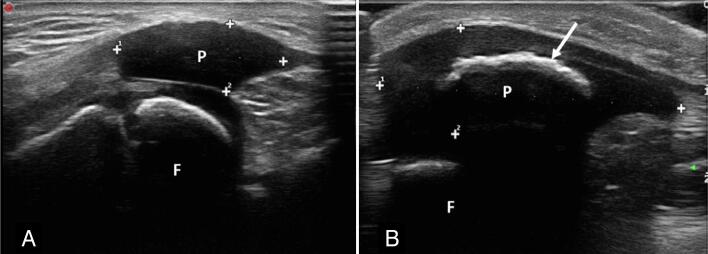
Male patient, aged 6 years and 9 months, with a previous diagnosis of right fibular hemimelia, complete agenesis of the fibula, and shortening of the ipsilateral femur and tibia. The right patella (A) was not easily identifiable on physical examination. The longitudinal ultrasound image shows patellar hypoplasia and absence of ossification, with the patella still cartilaginous, while the left patella (B) already shows ossification in the central region (arrow).
